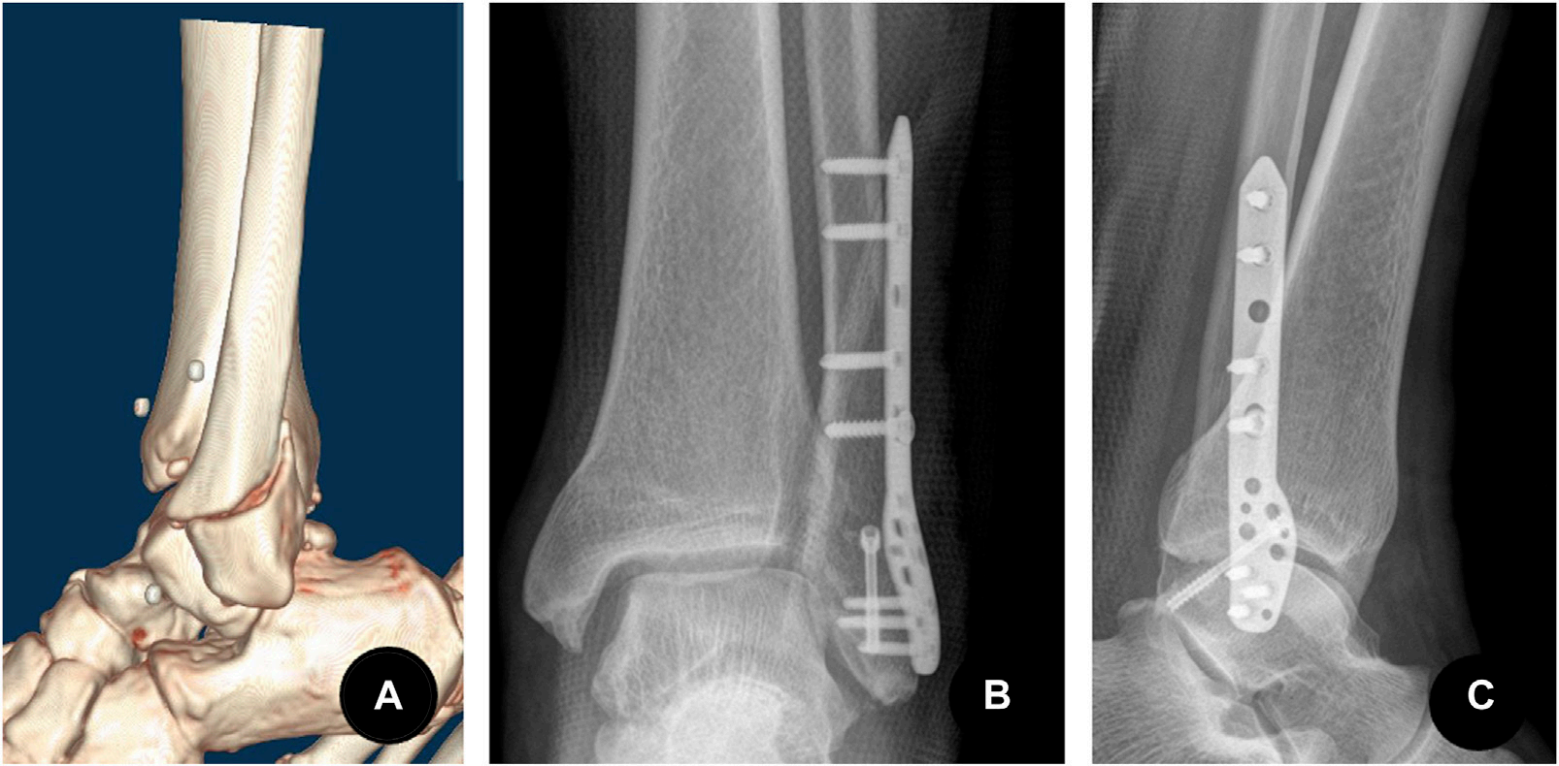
Type B lateral malleolar fracture fixed by a compression screw and a neutral plate mode that is applied on the lateral side. (A) A 57-year-old male patient with injury to the left lateral malleolus. Preoperative CT three-dimensional reconstruction showed a type B spiral fracture of the lateral malleolus, the distal fragment moved backward and upward. (B) The postoperative anteroposterior X-ray showed the lateral malleolus was fixed with a compression screw crossing the fracture lines and a lateral plate. The lateral plate acted as a neutralizing plate to protect the compression screw.(C) Postoperative lateral X-ray.
